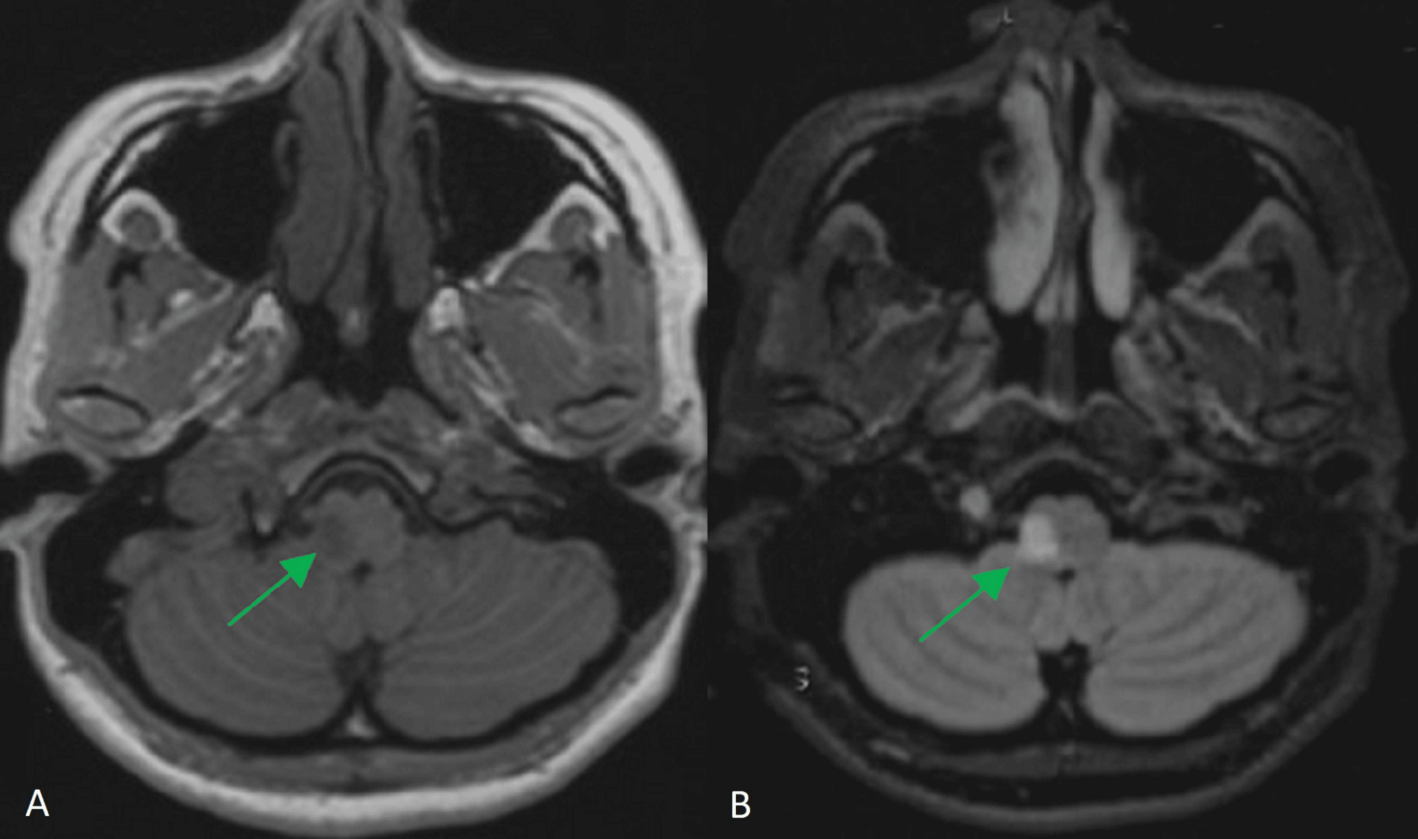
(A) T1-weighted and (B) T2-weighted axial MRI sequences showing a focal infarct in the right lateral medulla oblongata (ventrolateral region). MRI, magnetic resonance imaging.
